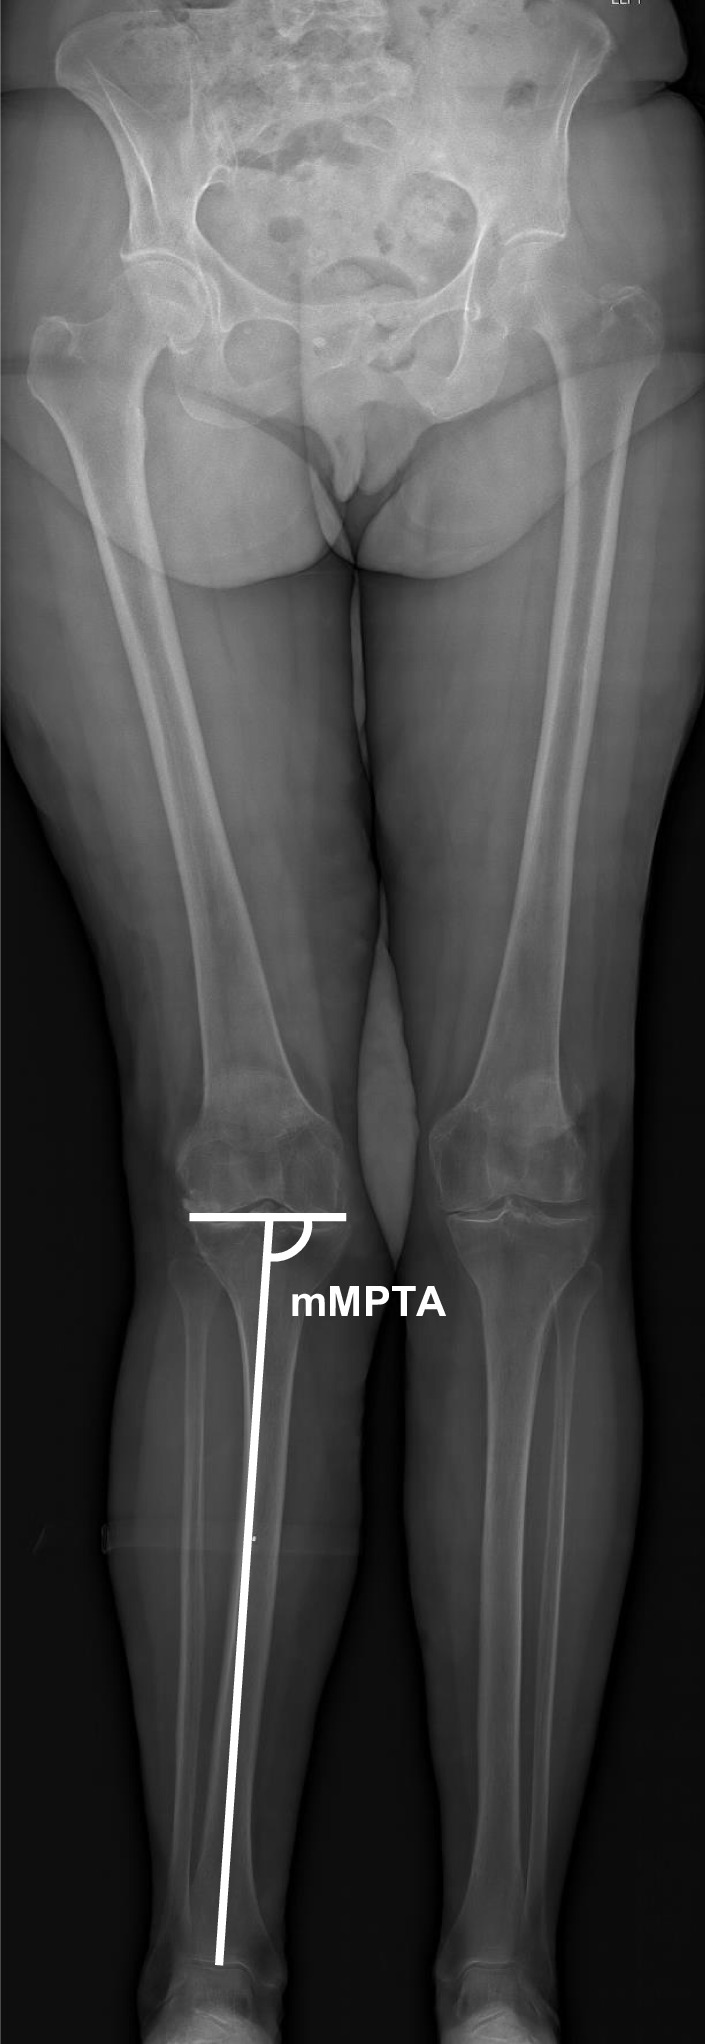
Mechanical medial proximal tibial angle (mMPTA). Pre-operative weightbearing lower-limb anteroposterior EOS digital radiograph is shown. The mLDFA is the angle between the mechanical axis of the femur and its articular surface
